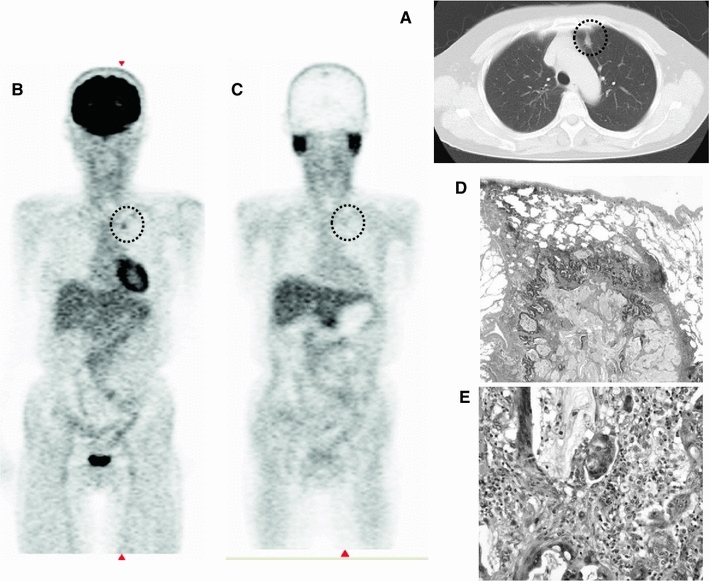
A case of metastatic colon cancer. Sixty-two year-old asymptomatic female patient and without elevated tumor markers. She had been followed as an outpatient after the resection of primary advanced colon adenocarcinoma with mucin secretion. In the follow-up period (1 year after surgery), the patient underwent a contrast-enhanced chest CT scan (a), which revealed a small lung nodule in the left apex. Both ^18^FDG and ^11^C-MeAIB PET showed faint accumulation in the left upper lobe lesion (SUVmax = 3.12 for ^18^F-FDG, 2.06 for ^11^C-MeAIB). Both ^18^F-FDG and ^11^C-MeAIB PET diagnoses were “equivocal” (visual diagnosis: negative, quantitative diagnosis: positive) (b, c). After close follow-up by CT scan and tumor markers, she underwent left upper lobe resection, and this lesion was confirmed as metastatic colon cancer with mucin secretion by pathology (d: low magnification, e: high magnification)
